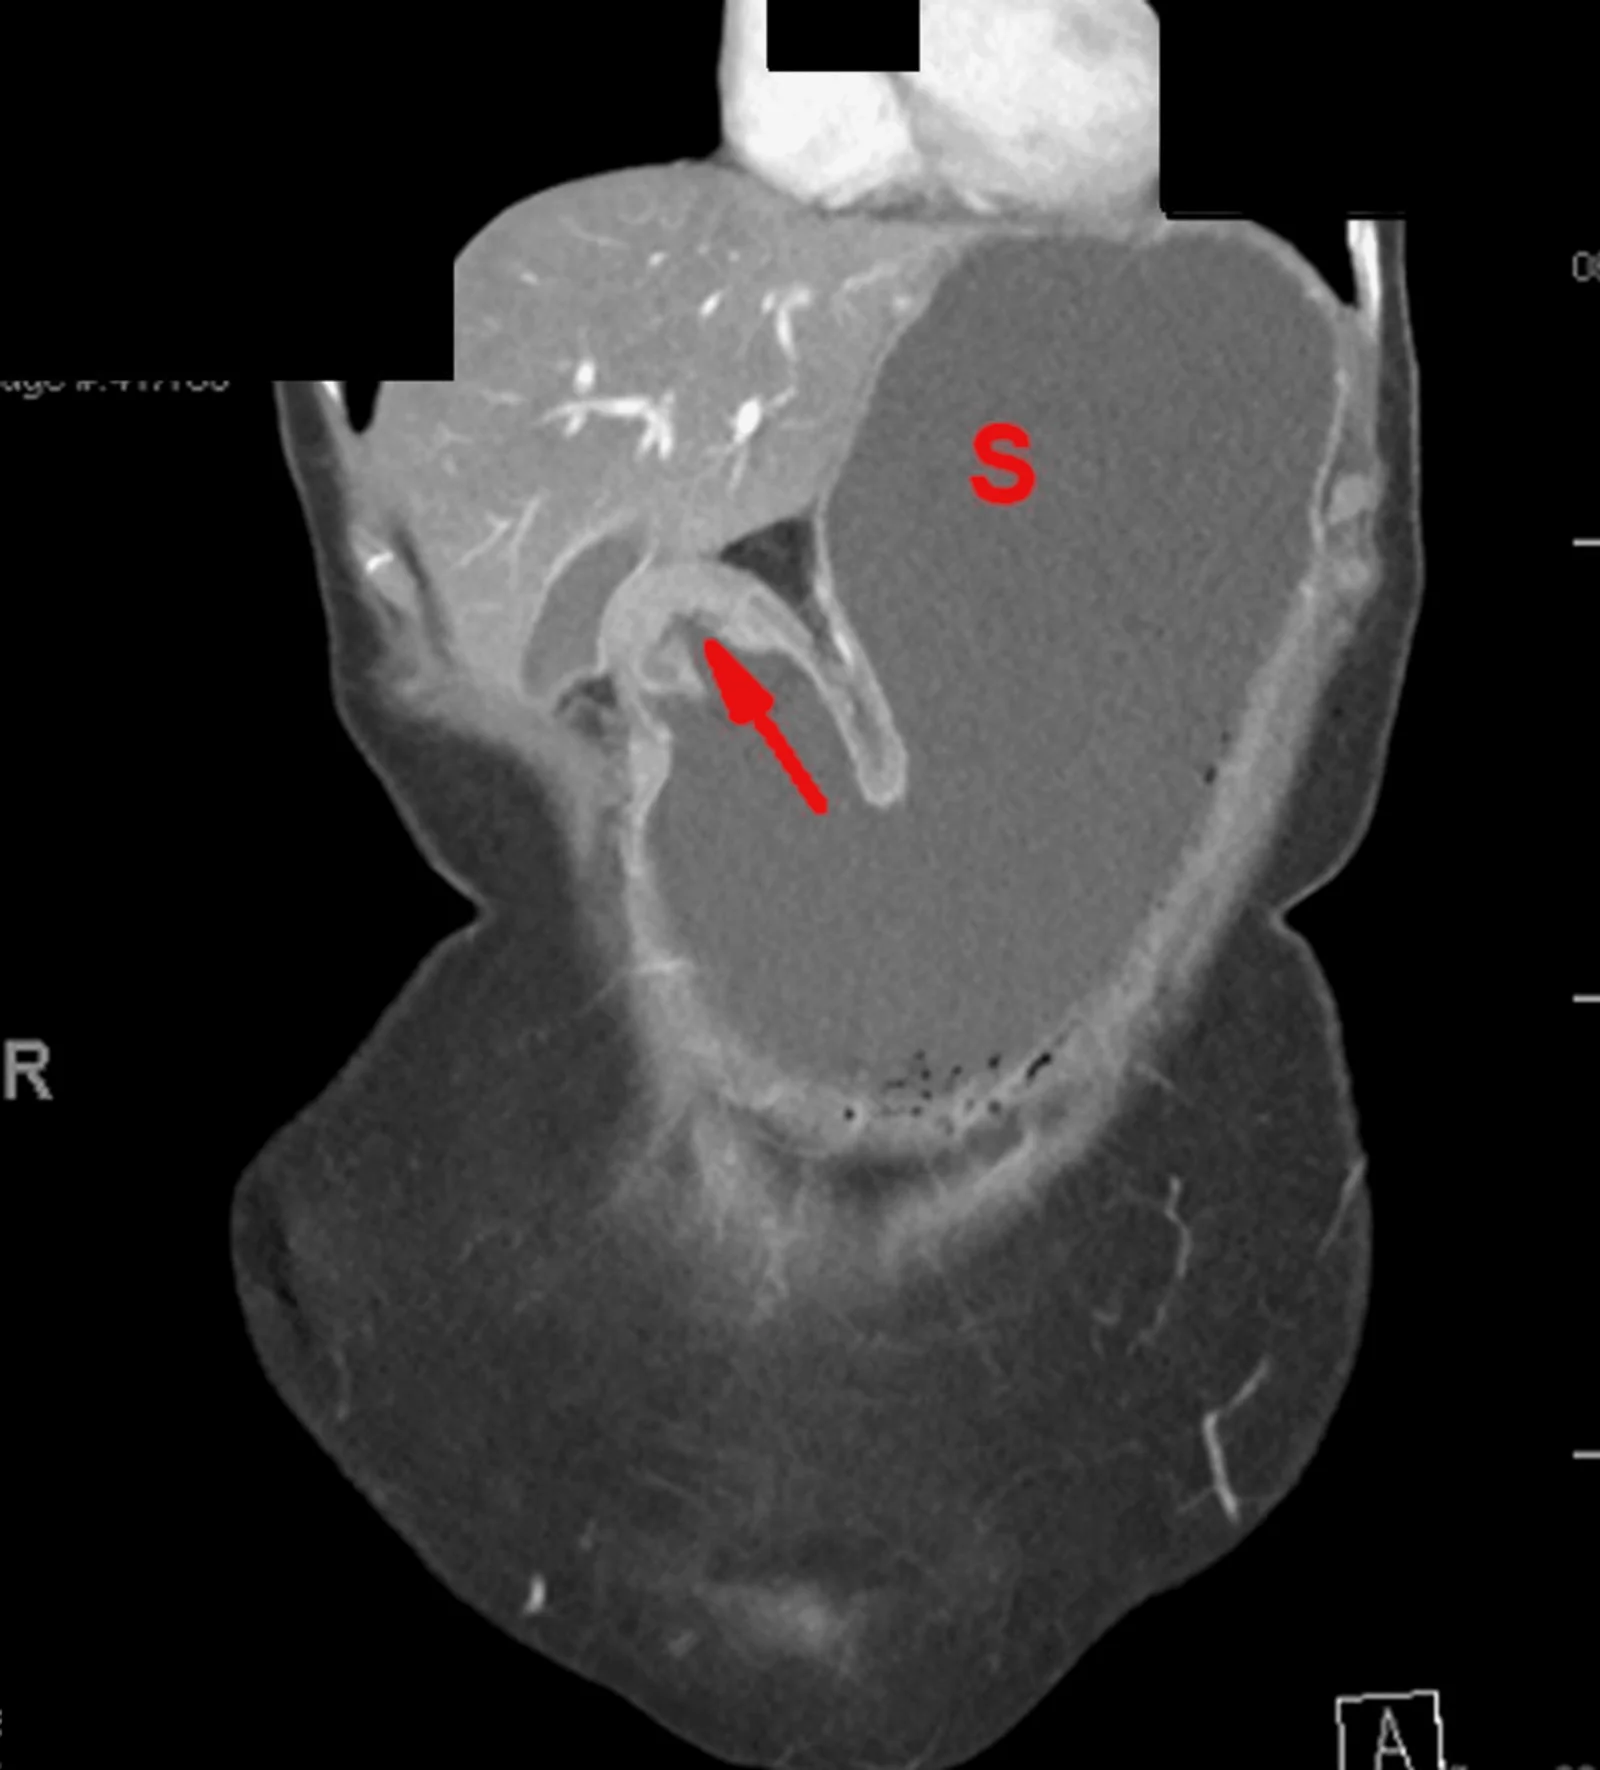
CT with contrast of the abdomen and pelvis. ‘S’ denotes the enlarged stomach. The arrow shows the region of outlet obstruction.
